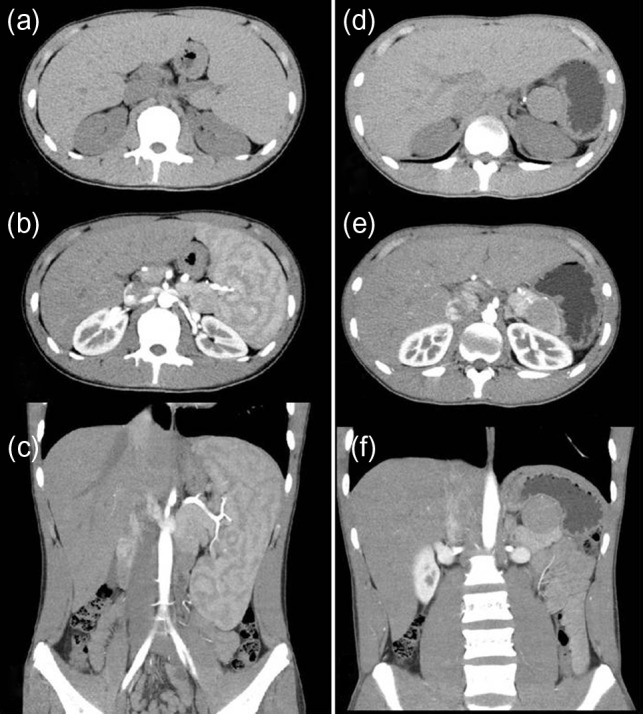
Computed tomography (CT) (before and after splenectomy). (a–c) Before splenectomy: Plain and enhanced CT shows a huge spleen and a 2-cm mass near or in the pancreatic tail. (d–f) After splenectomy: Plain and enhanced CT shows the mass in the pancreatic tail grows rapidly to 4 cm.
